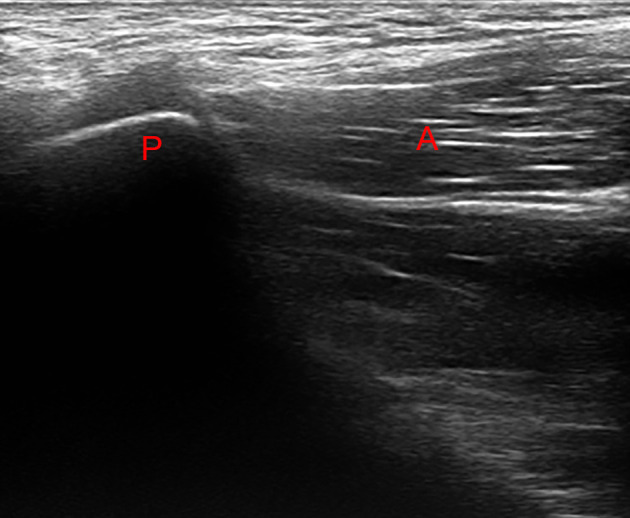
Ultrasound investigation showing pubic insertion of the adductor musculature. No evidence of functional overload is present.
